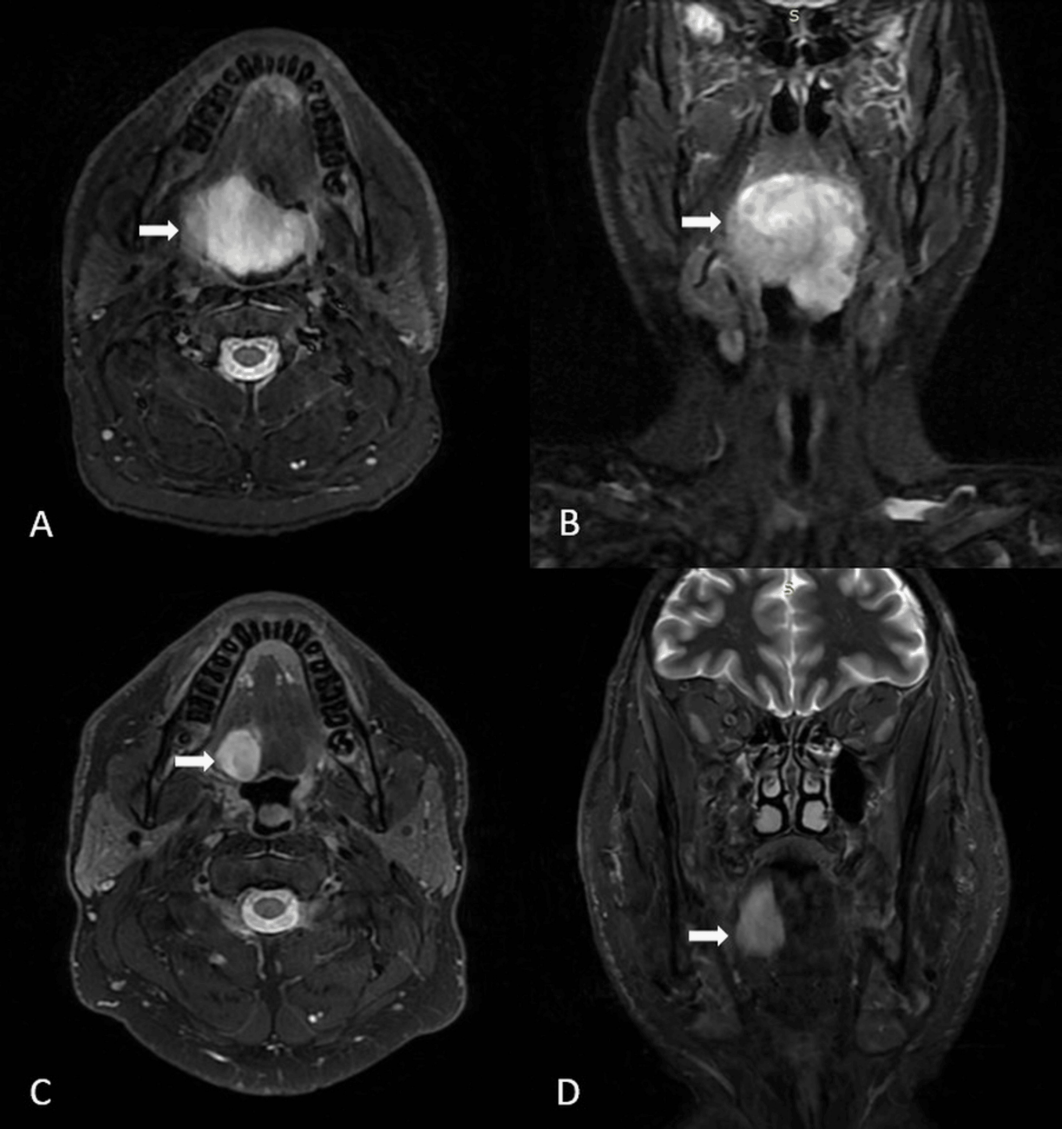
Imaging findings (white arrow showing the lesion) A,B) Axial and coronal T2-weighted MRI showing a preoperatively hyperintense lesion at the tongue base, extending laterally. C,D) Axial and coronal T2-weighted MRI revealing a recurrent lesion on the right lateral aspect of the tongue.
